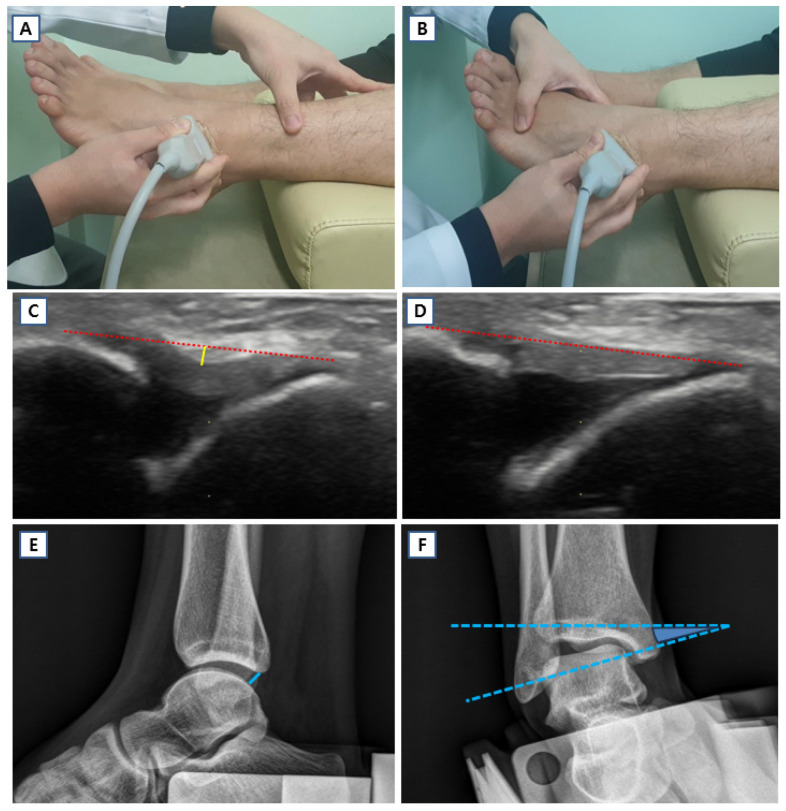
Stress ultrasonography and stress radiography of the ankle. Ultrasound images of the anterior talofibular ligament (ATFL) were obtained in (A) the resting position and (B) during maximal plantar flexion and inversion stress. Representative images from a 21-year-old dancer with a Beighton score of 7 are shown in (C) the resting position and (D) during stress. ATFL length (dotted red line) was defined as the linear distance between the talar and fibular attachment sites, and ATFL height (yellow line) was measured as the maximum perpendicular distance from the length line to the superficial border of the ATFL, representing ligament laxity. For stress radiography, (E) anterior drawer stress and (F) inversion stress were applied using a force of 150 N. Anterior talar translation (blue line) was measured as the distance between the posterior lip of the tibia and the nearest articular surface of the talus, and talar tilt was defined as the angle (blue angle) between reference lines drawn along the talar dome and the tibial plafond.
